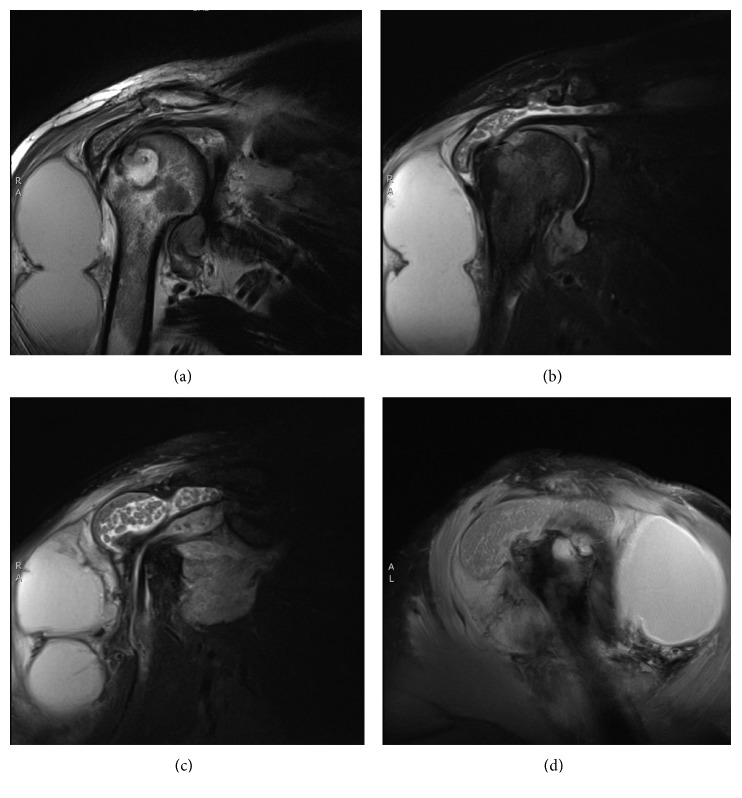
MRI of the right shoulder showing large subacromial subdeltoid fluid collection with the so-called rice bodies. A large extra-articular fluid collection is seen possibly communicating with the subacromial subdeltoid bursa.
